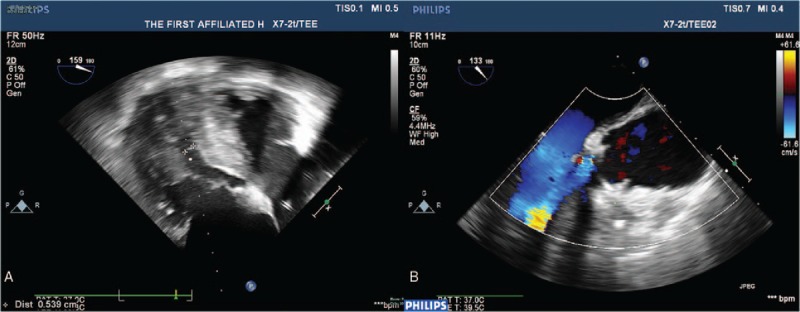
(A) The intraoperative transoesophageal echocardiogram before second surgery. There was a severe aortic regurgitation. (B) The intraoperative transoesophageal echocardiogram before second surgery. The left ventricle outflow tract was as narrow as 0.539 cm owing to the bulged septum.
